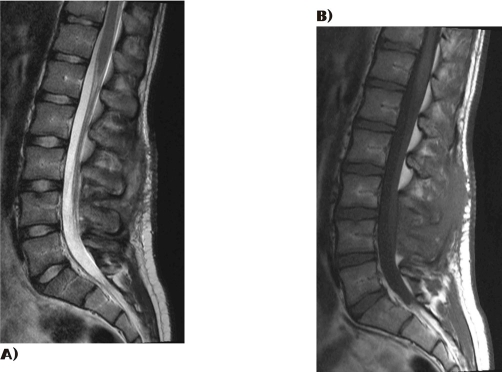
MRI scan show the final result after the decompression of the hematoma at two months.
